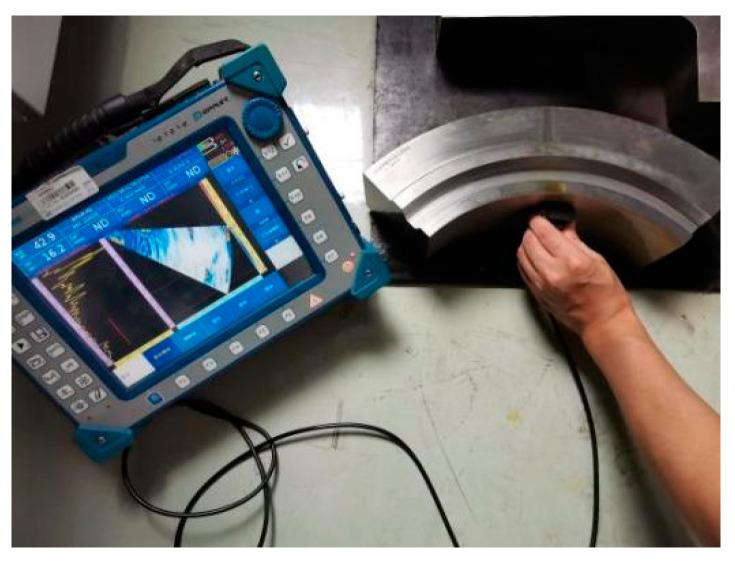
Ultrasonic phased array field test.
